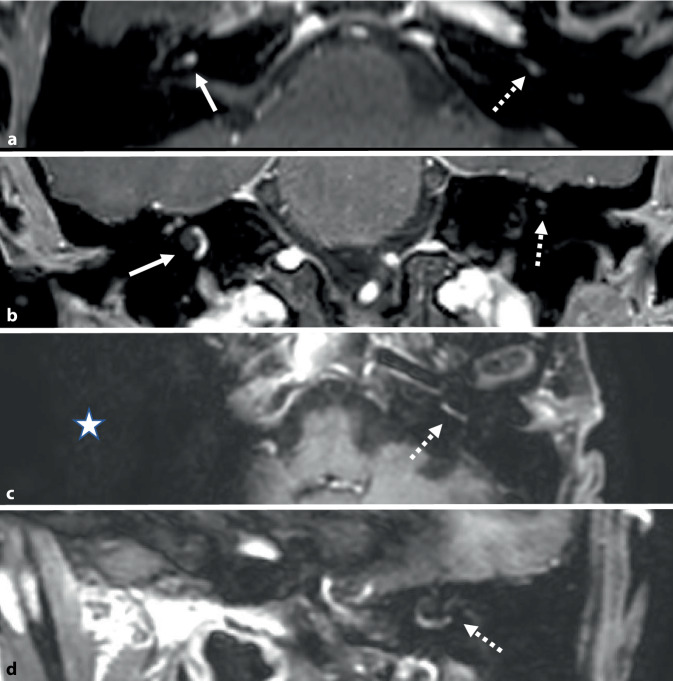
Intracochlear schwannomas (ICS) identified on axial and coronal gadolinium (Gd)-enhanced T1-weighted magnetic resonance imaging (MRI; Philips DRIVE T3 MRI scanner). Enhancing right ICS (*solid arrows*) on axial (a) and coronal (b) and early small left ICS (*dashed arrows*) prior to right-sided surgical intervention. Progressive growth of left-sided ICS (*dashed arrows*) on axial (c) and coronal (d) repeat MRI after right-sided surgery with artifact (*star*) from cochlear implant
